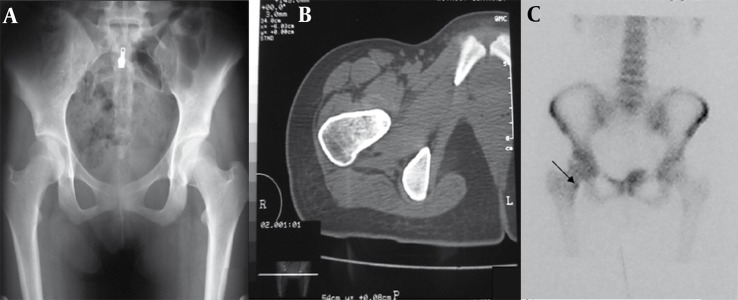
A 20-Year-Old Woman Presented With a One-Year Right Hip Pain. A, Plain radiograph of the hip revealed no abnormalities. B, CT scan of the hip shows a sclerotic lesion with a central nidus in the cortex. C, In radionuclide scan an increased uptake was detected in the same region (arrow).
